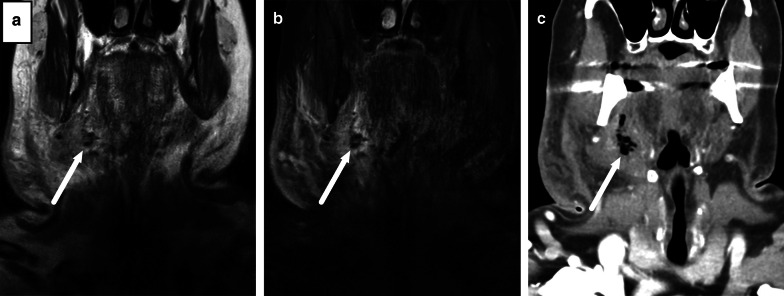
On MRI, gas appears as small foci of signal void (arrows) on coronal in-phase (a) and fat-suppressed T2-weighted (b) images but is easier to see on CT (arrow) (c)
